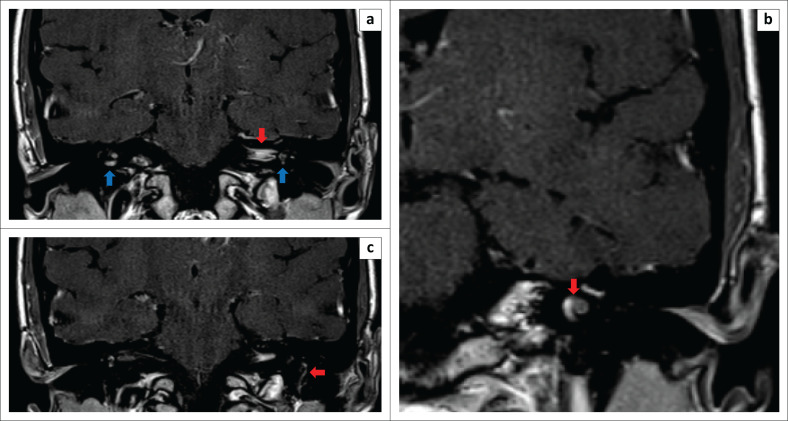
(a) Coronal, T1 weighted post contrast MRI demonstrated enhancement in left internal acoustic canal and facial nerve (red arrow) as well as bilateral basal turns of the cochleae (blue arrows). (b) Coronal, T1 weighted post contrast MRI demonstrated enhancement of bilateral basal turns of the cochleae (only left side shown, indicated by red arrow). (c) Coronal, T1 weighted post contrast MRI demonstrated enhancement of the left 7th cranial nerve vertical segment with a nodular appearance (red arrow).
